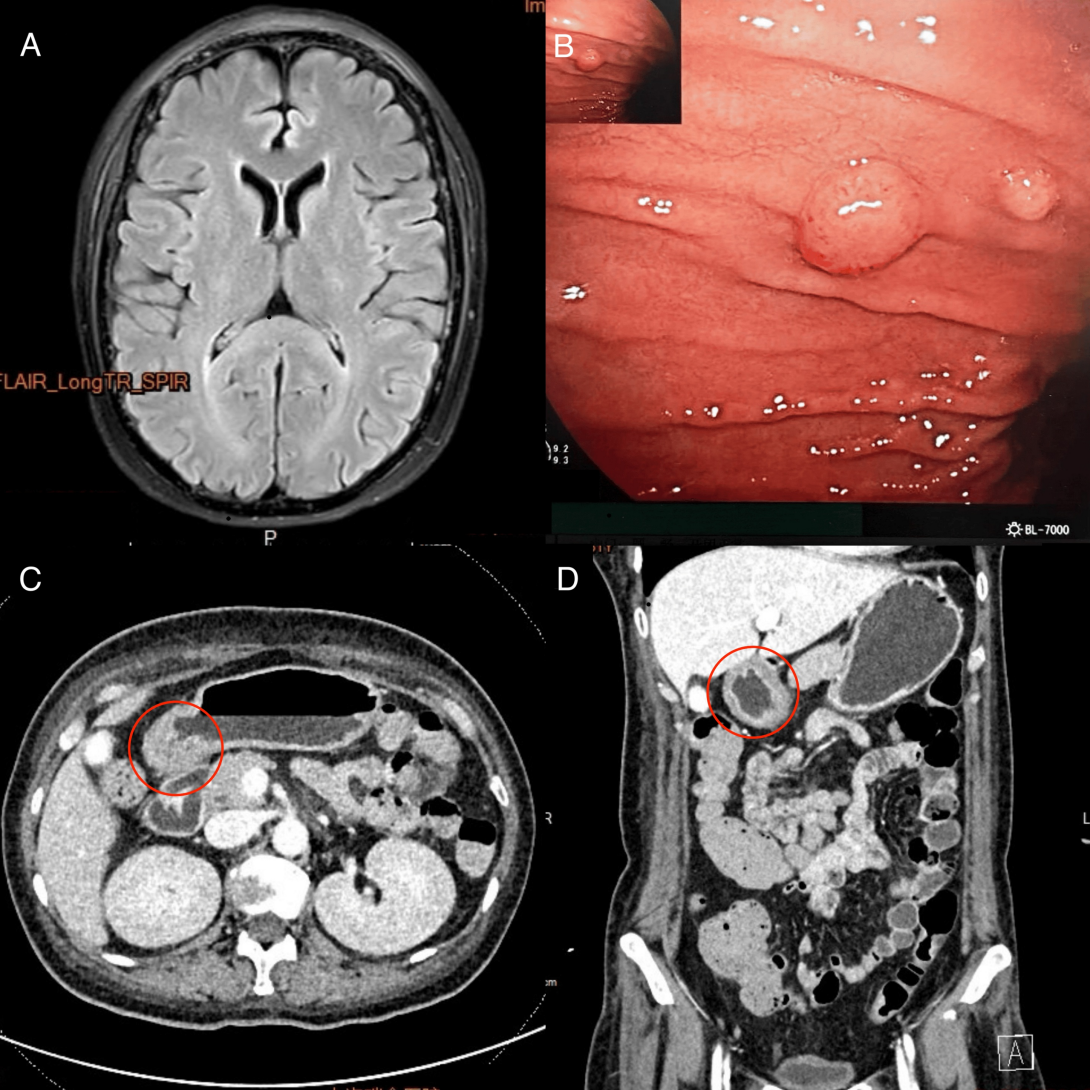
The MRI image of the brain, gastroscopy, and contrast-enhanced CT of the small intestine A: Brain MRI (without contrast) showing no significant abnormalities and no meningeal signs. B: Gastric body and fundus: smooth mucosal folds. A polypoid lesion measuring approximately 1 cm was observed on the greater curvature near the anterior wall of the mid-body, along with multiple broad-based polyps. C-D: CT of the small intestine (contrast-enhanced, non-ionic contrast agent (100 mL) was injected via the antecubital vein at 3 mL/s) after oral mannitol showed thickening of the gastric antrum with a layered hypodense area in the submucosa.
